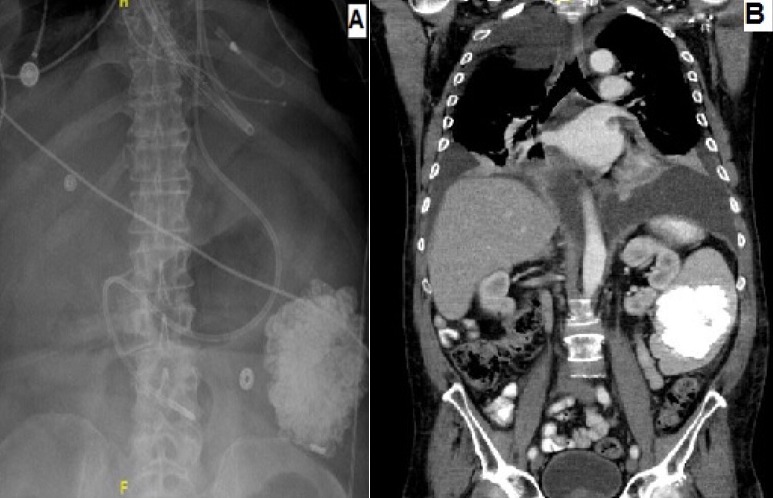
A) abdominal X-ray showing massive intra-peritoneal calcification; B) CT abdomen, showing extensive splenic calcification
